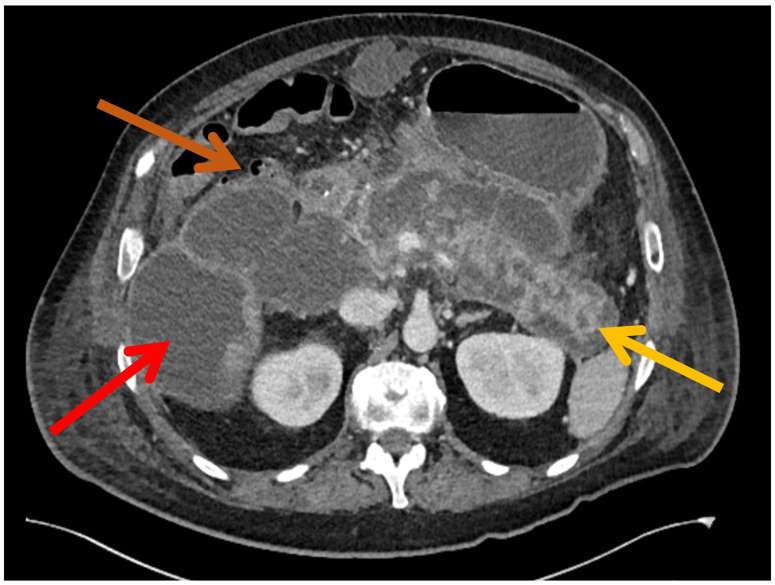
Diagnosis of WON in CT. Red: WON; yellow: pancreas; brown: colon.
